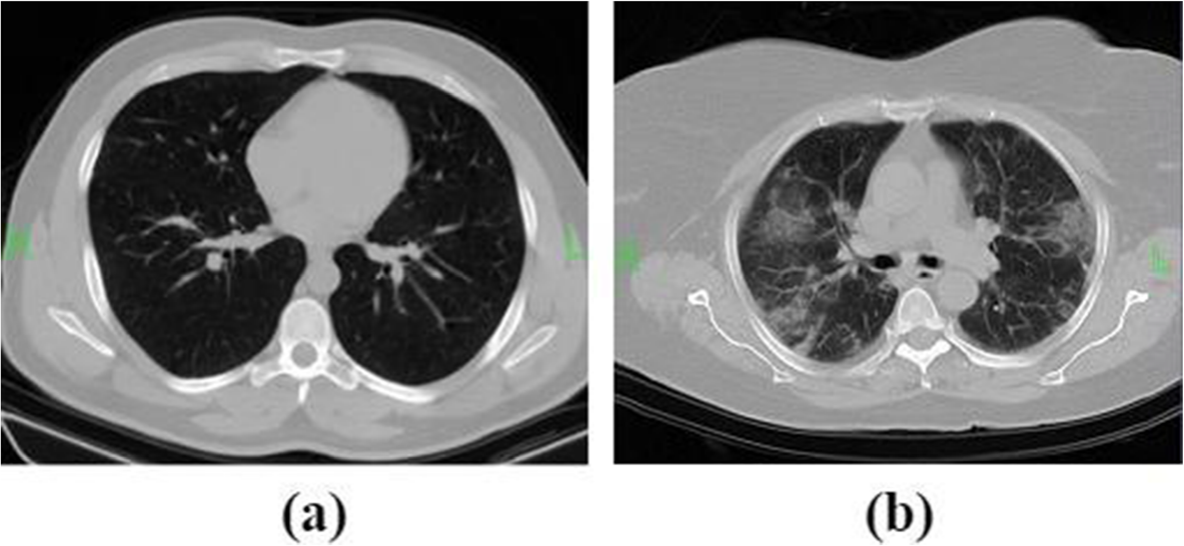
Sample of CT images from patients with suspected COVID-19 (a) and confirmed (b)
